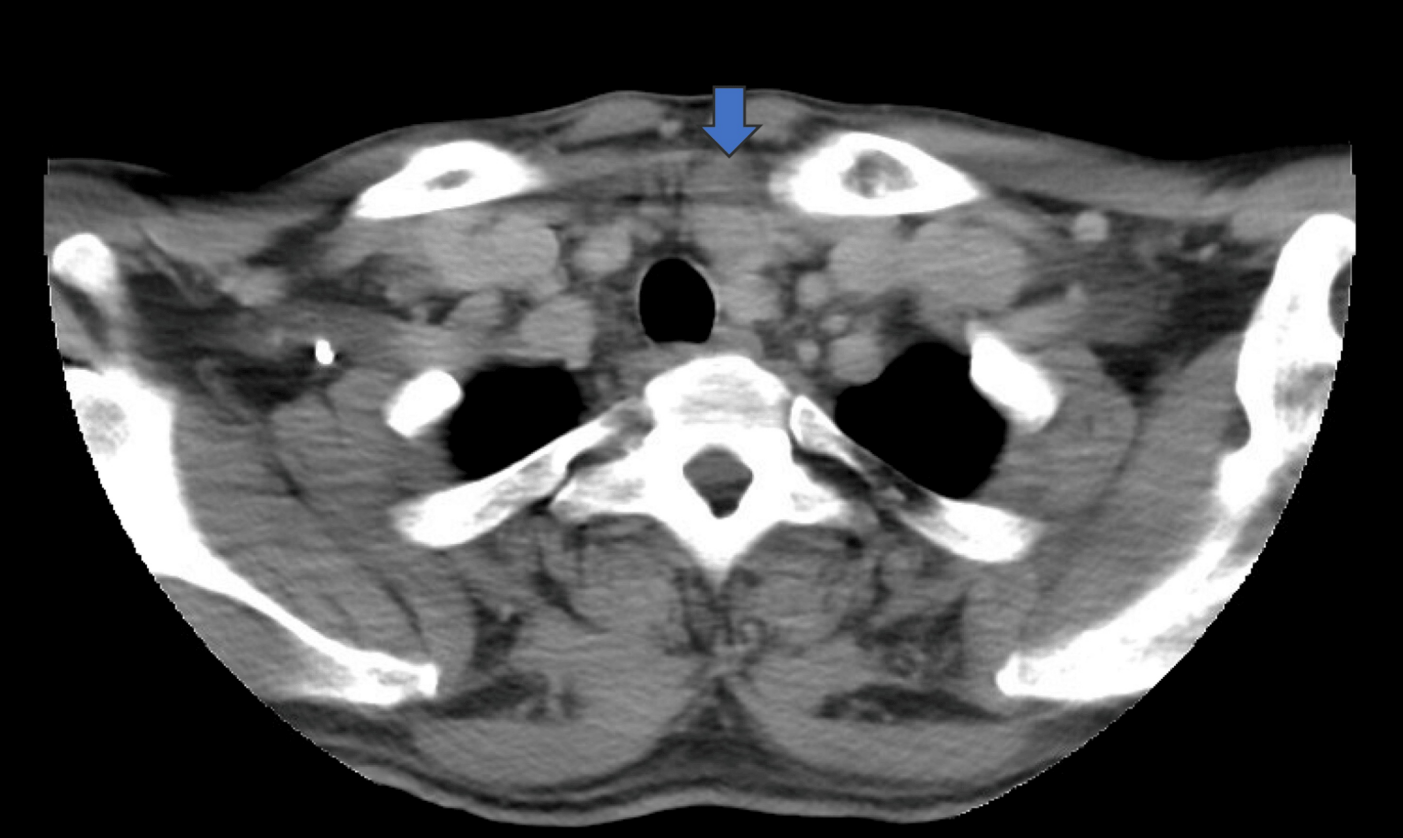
CT of the neck showed a massive goiter with a 2.1 cm exophytic nodule at the lower pole of the left thyroid lobe
